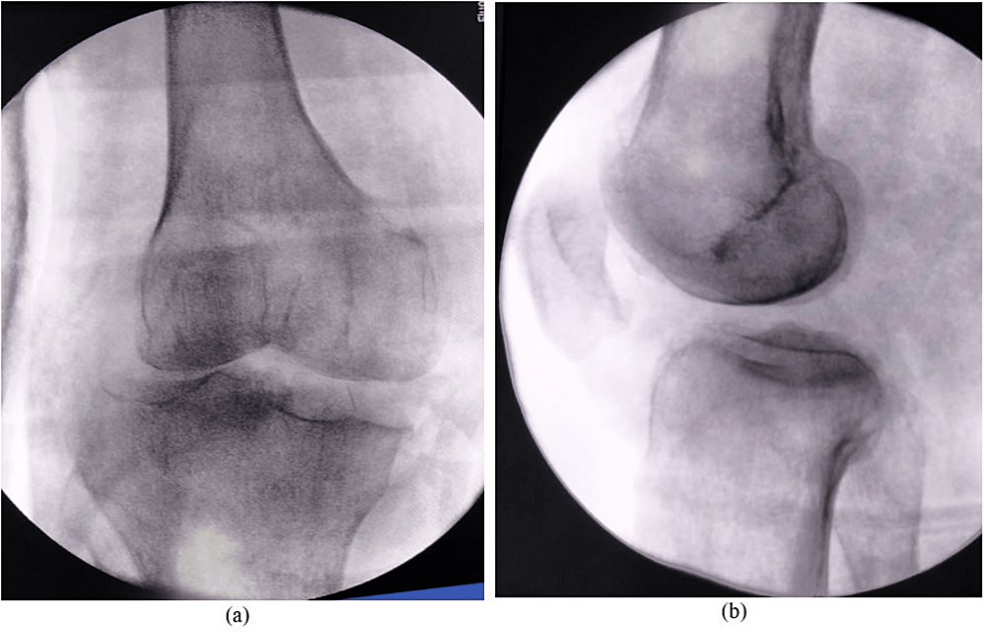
Anteroposterior and lateral radiographs showing posterolateral subluxation and medial tibiofemoral compartment widening.
